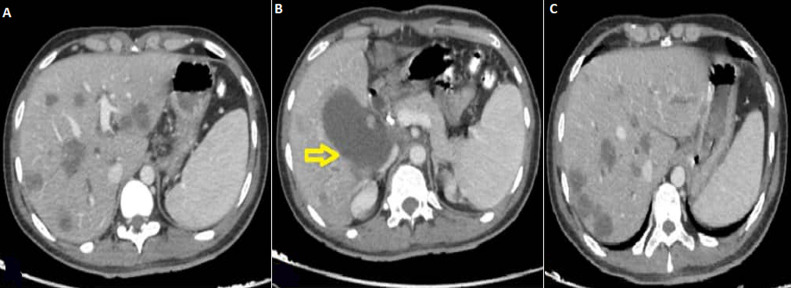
A) changes in metastatic liver lesions evaluated by computed tomography during chemotherapy at five months; B) abdominal computed tomography scan showing common bile duct dilatation (arrow); C) follow-up abdominal computed tomography at nine months showing no regression in mass size
